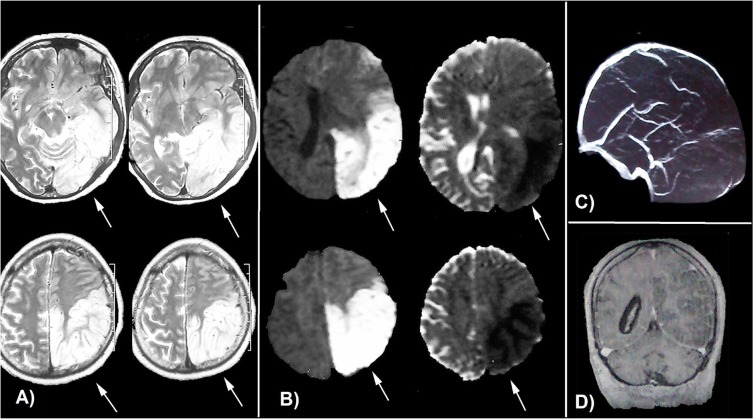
A) Axial t2-weighted brain MRI; hypersignaling in left parietooccipital lobes with mass effect; B) Axial diffusion-weighted MRI and ADC show water restriction in left parietooccipital lobes related to acute ischemia; C) Brain MRV shows no abnormality in dural sinuses; D) Coronal t1-weighted brain MRI with contrast injection; hypo-signality in left parietooccipital lobes with mass effect on lateral ventricle without contrast enhancement. (ADC: Apparent Diffusion Coefficient; MRV: Magnetic Resonance Venography)
